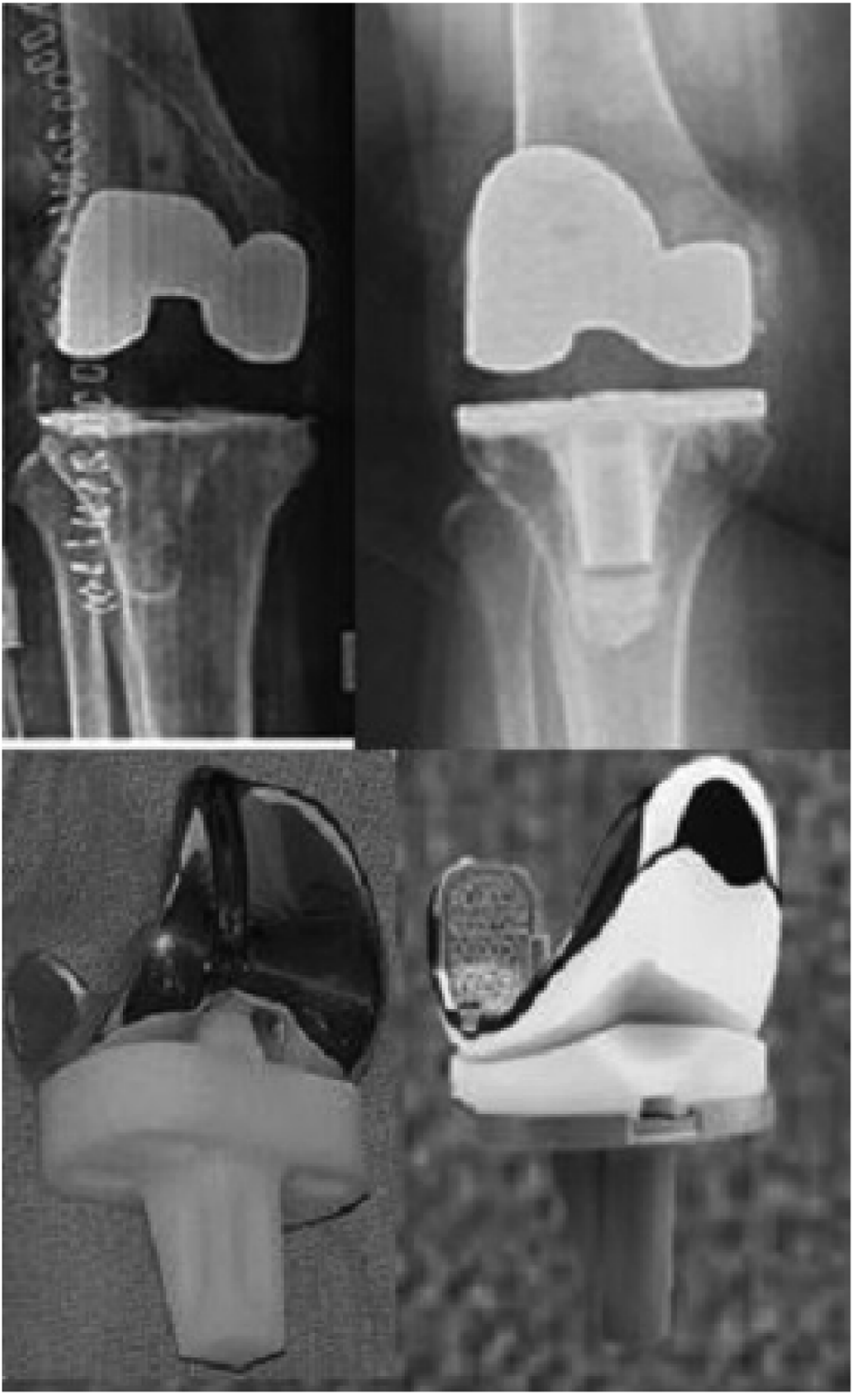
Comparison of both implants as well as their appearance on plain radiographs. All-poly tibia is radiolucent (the left part of the figure) while both have metal backed femoral implant.
